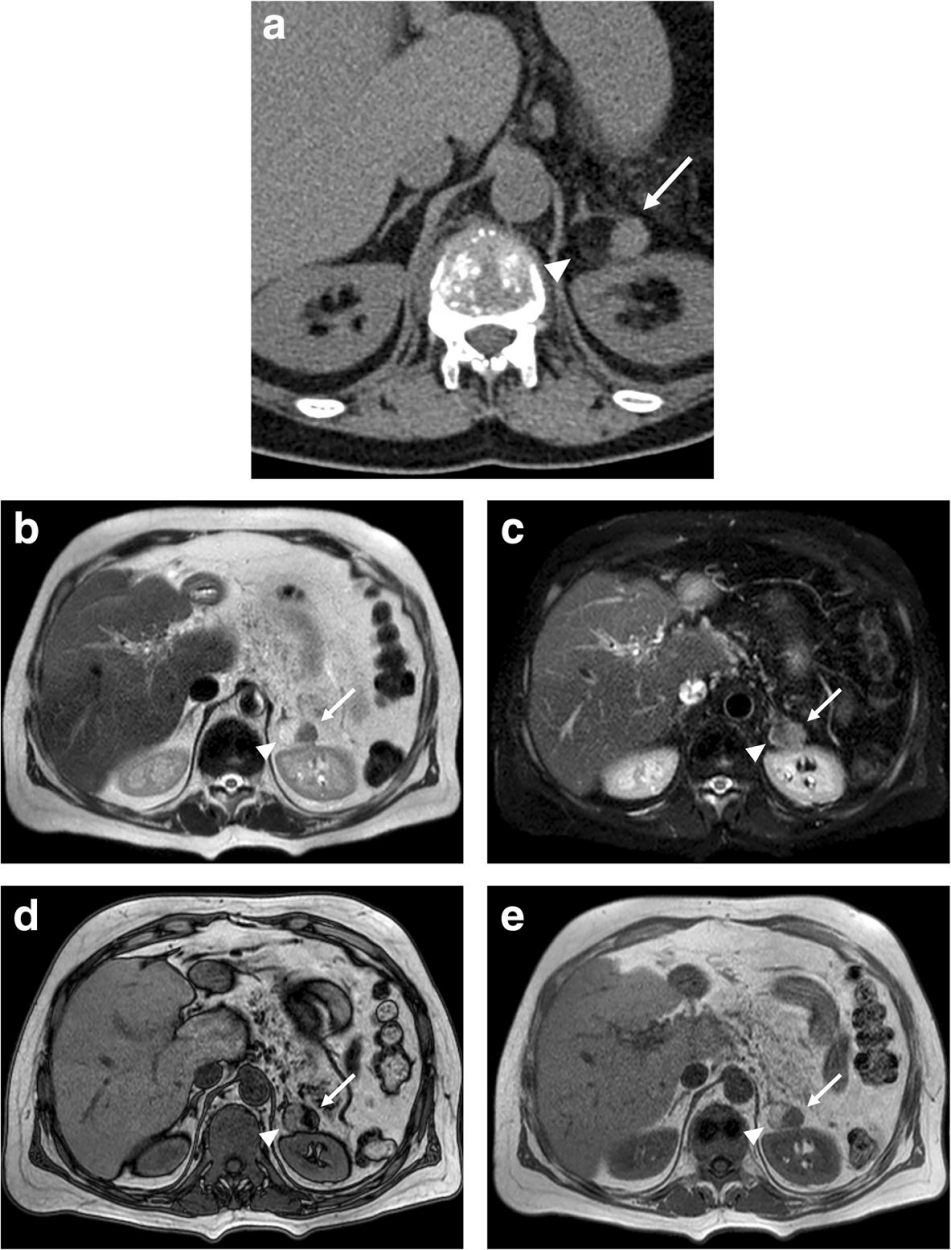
Left adrenal myelolipoma incidentally discovered in a lumbar CT scan on a 51-year-old man. Unenhanced CT (a) shows a left adrenal mass with macroscopic fat (arrowhead) and a soft-tissue myeloid component (arrow). Axial T2-weighted fast spin echo (b), T2-weighted fat-saturated spin echo (c), T1-weighted in-phase (d), and out-of-phase gradient recalled echo images (e) show the signal drop of macroscopic fat in fat-suppressed T2-weigthed images (c, arrowhead) and show loss of signal on out-of-phase imaging of the myeloid element (e, arrow)
